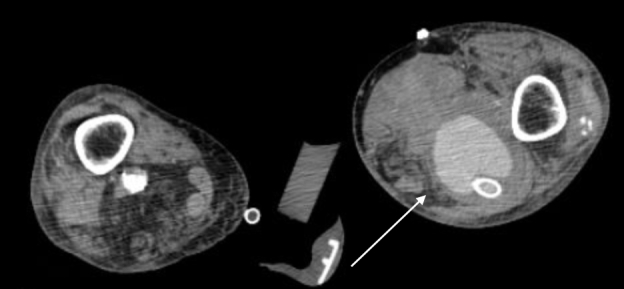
Computed tomography angiography of the left lower extremity in axial view demonstrating popliteal artery aneurysm extension into a large left lower leg hematoma (arrow) status post popliteal artery stenting and dermatofasciotomy.
